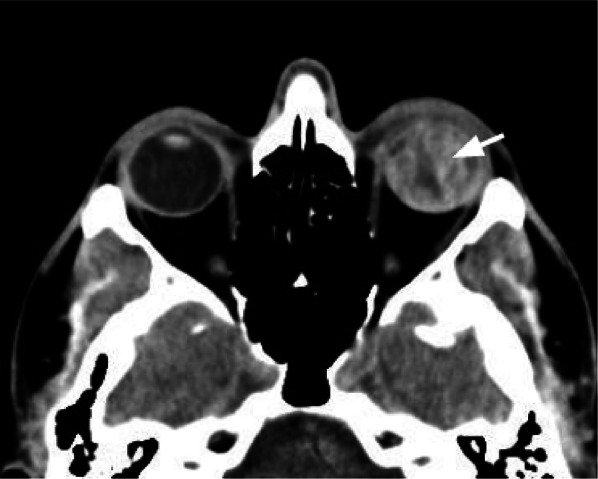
Axial CT acquisition. Left vitreous hemorrhage visible as a diffuse inhomogeneous hyperdensity of the left eyeball (arrow)
